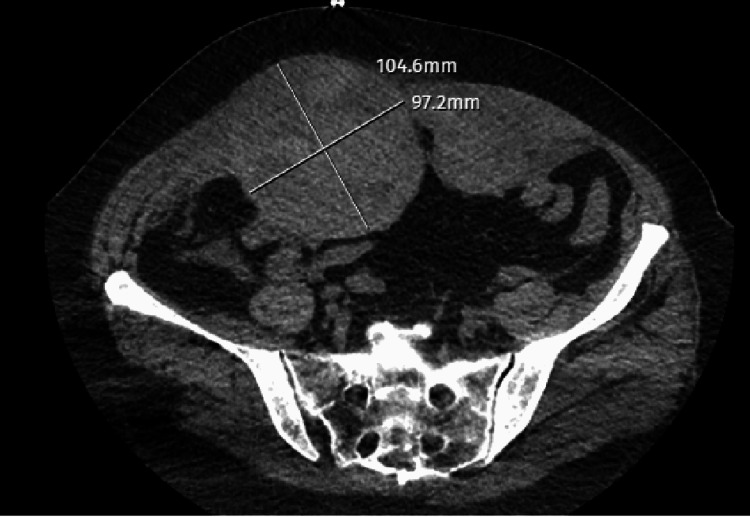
CT imaging of the hematoma CT: computed tomography
